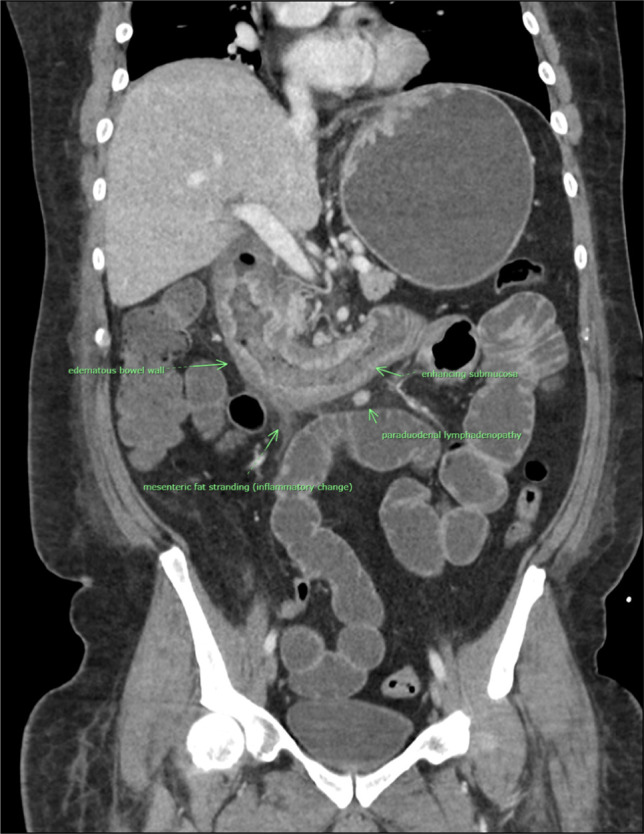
Abdominal and pelvic CT scan suggestive of small bowel enteritis.
